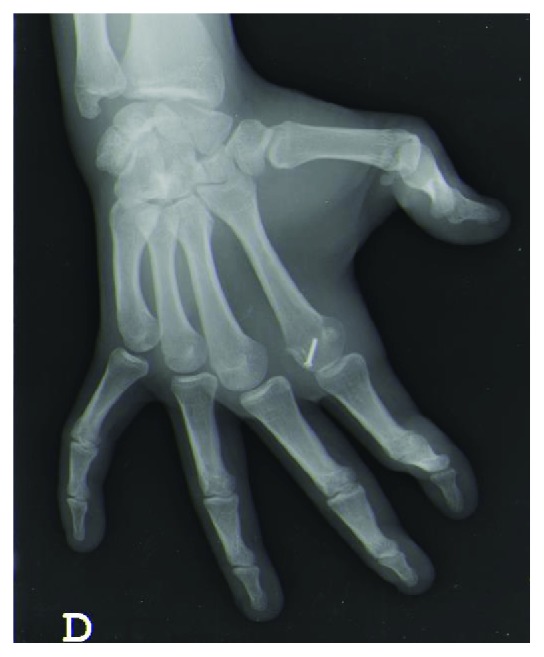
X-Ray (lateral view) showing fracture consolidation at week 6.
